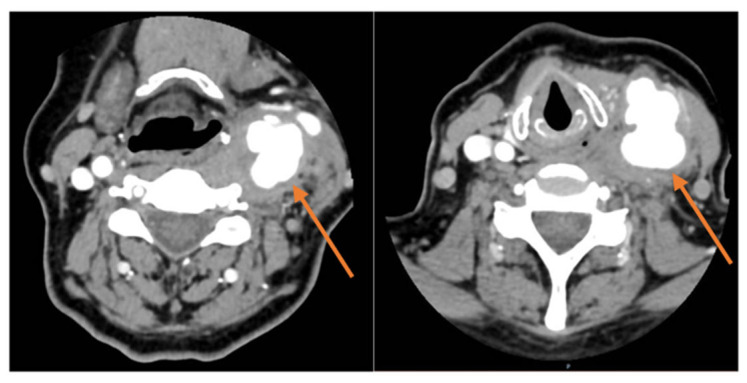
Giant left common carotid artery pseudoaneurysm on CT.
